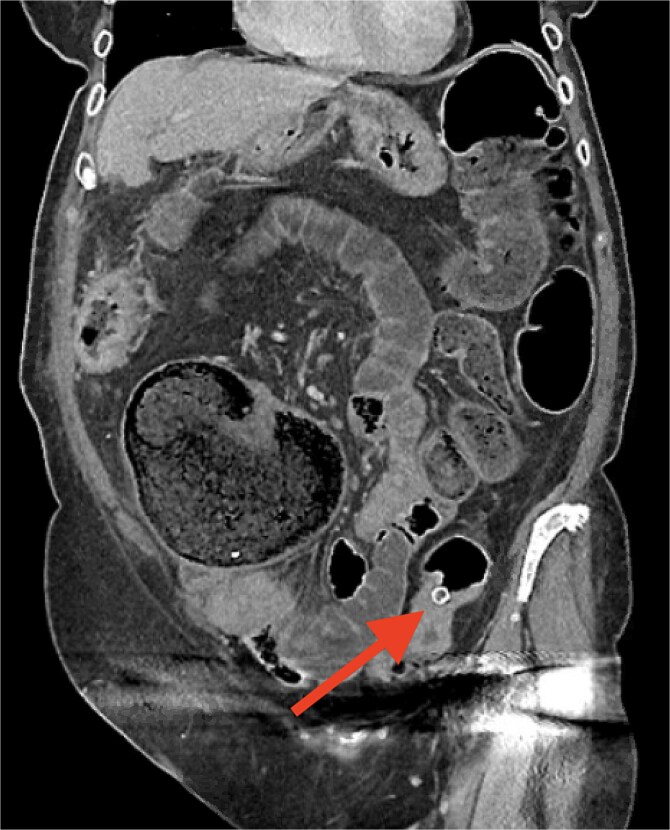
Subsequent CT demonstrating large bowel obstruction and migrated gallstone lodged at the distal descending colon. *This image was obtained at University of Oxford Hospital NHS Foundation Trust with patient consent.*
